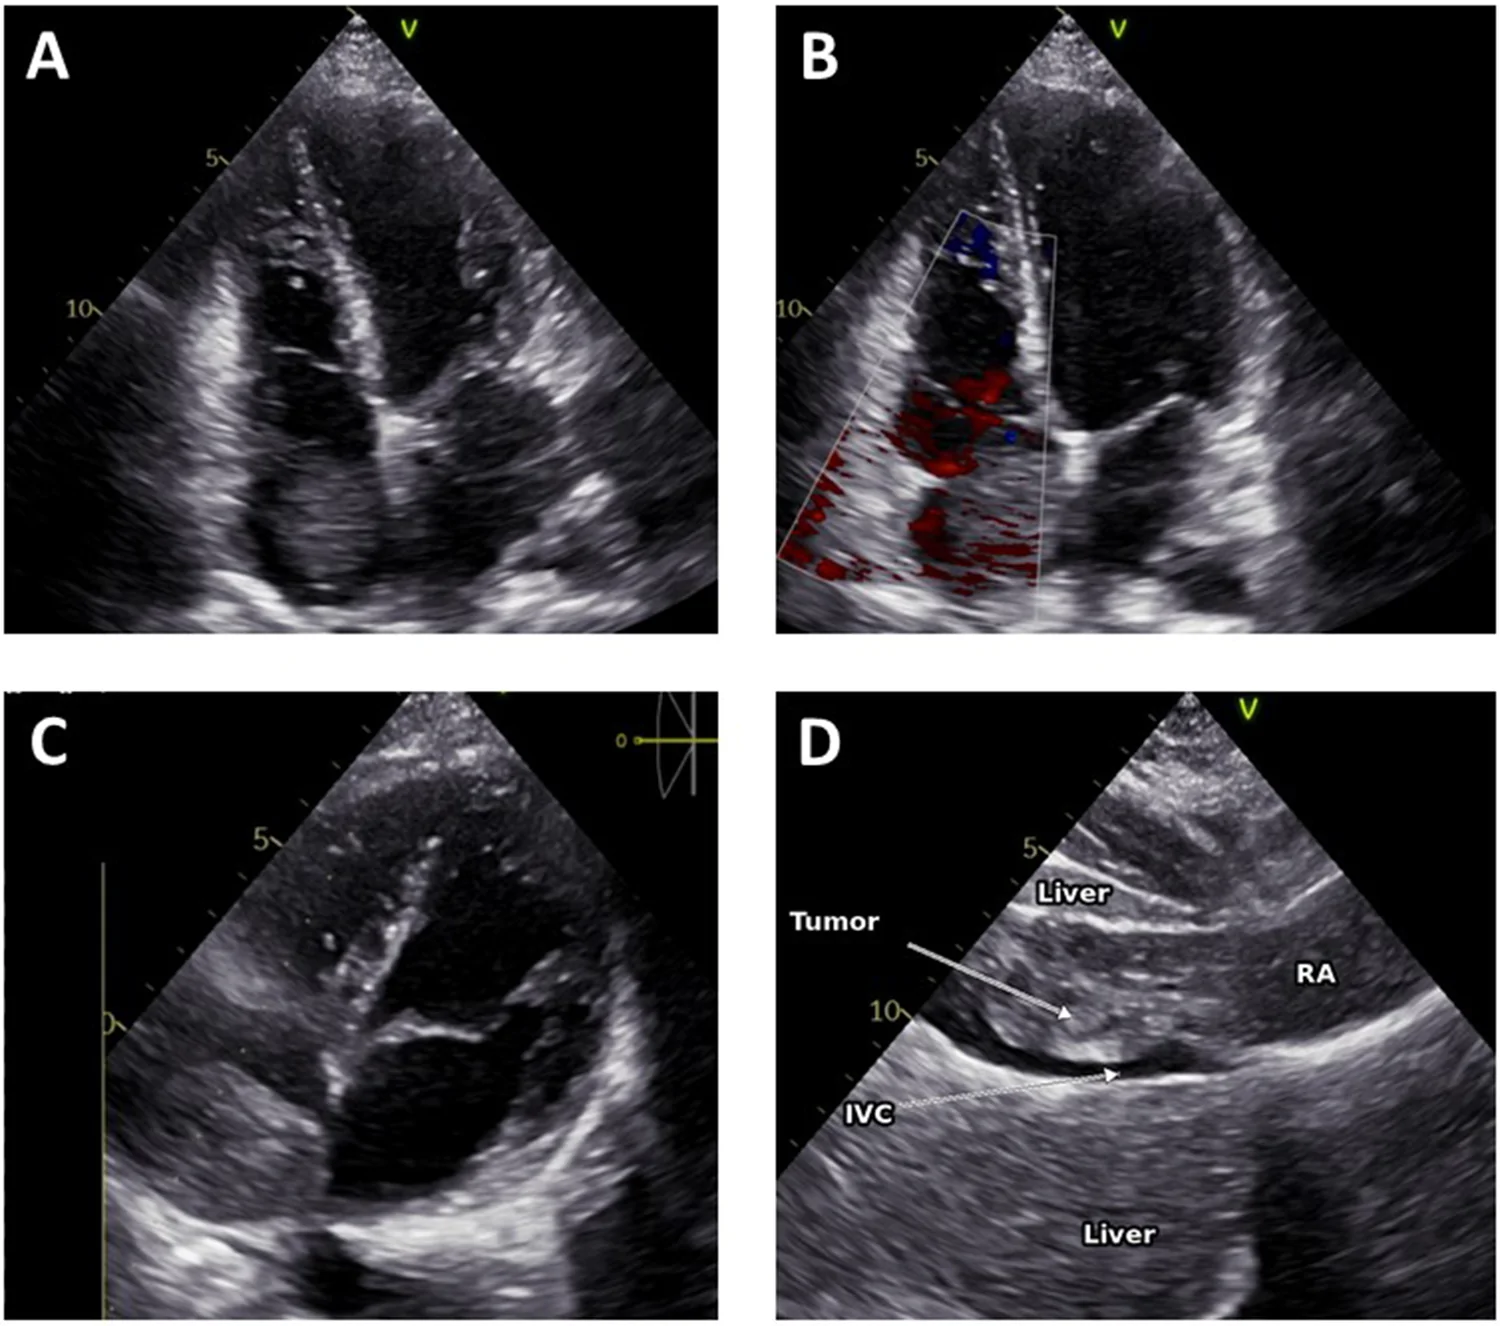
Transthoracic and transesophageal echocardiography demonstrating a right atrial mass. (A) Transthoracic echocardiography in four-chamber view showing a mass in the right atrium. (B) Color Doppler imaging demonstrating internal vascularity. (C) Transesophageal echocardiography showing detailed visualization of the tumor in the right atrium. (D) Subcostal view showing the mass at the junction between the inferior vena cava and the right atrium. IVC, inferior vena cava; RA, right atrium.
